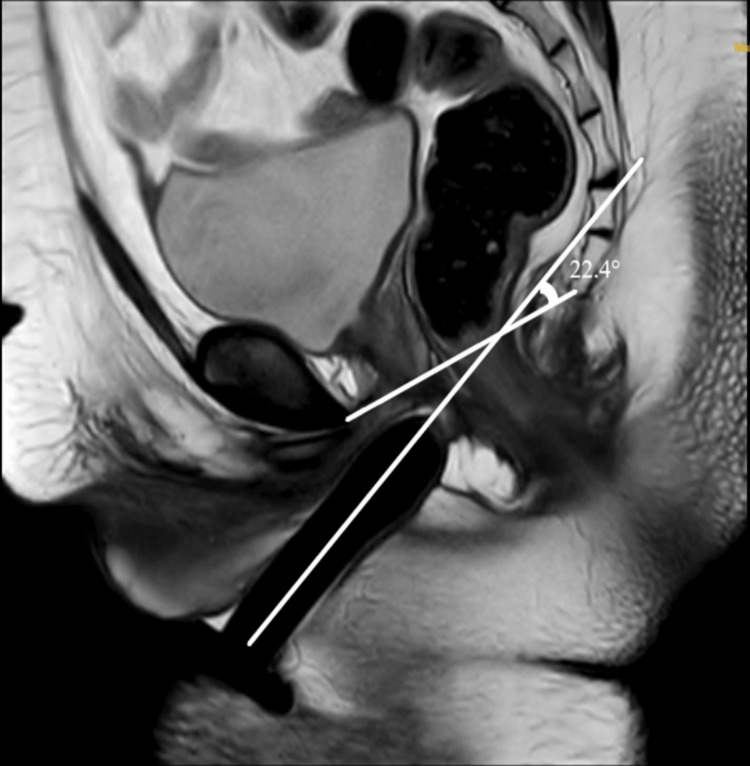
T2W HR sagittal image of a defaulter patient Original MRI image of a patient who underwent the study.
